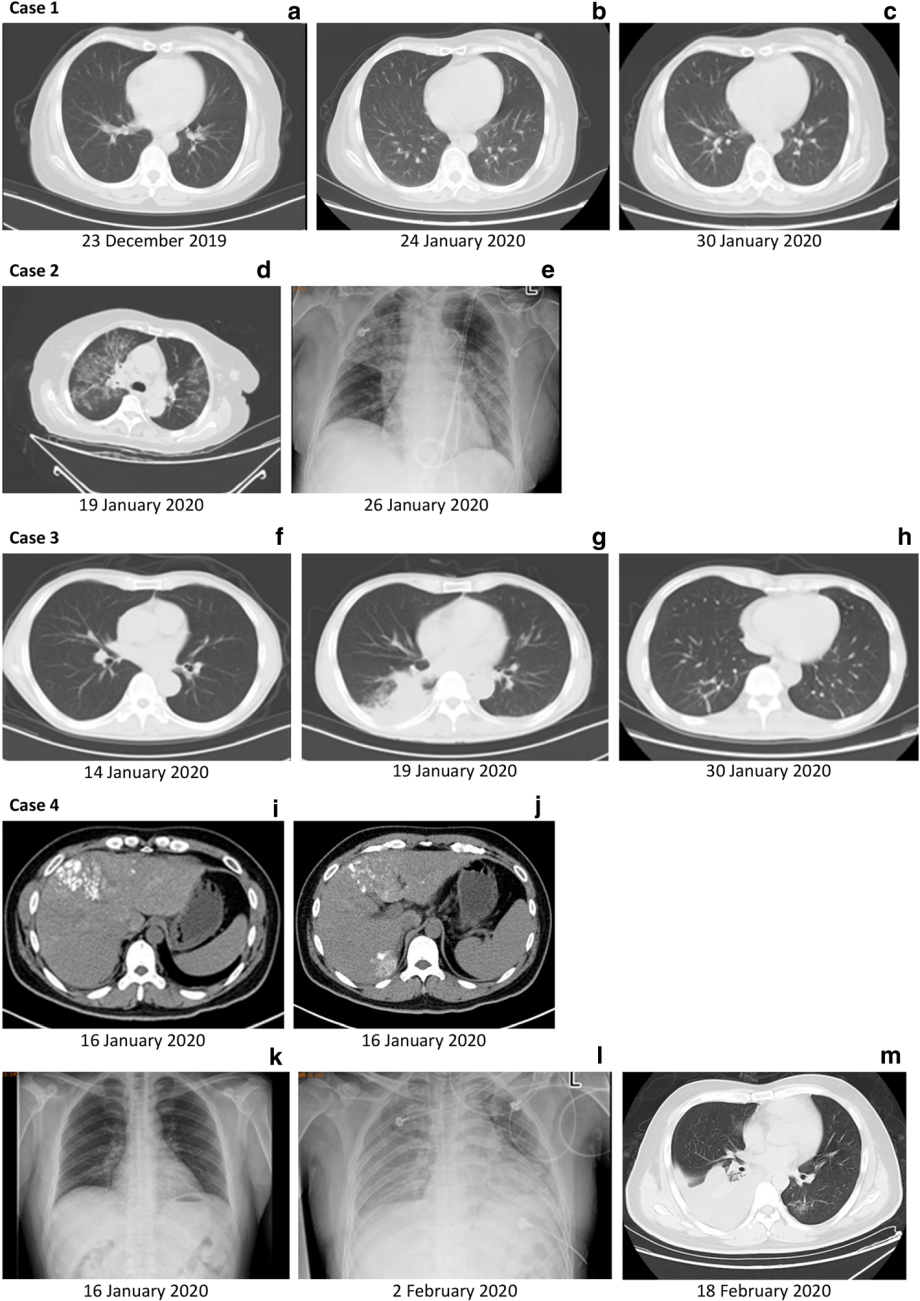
The chest X-ray/computed tomographic (CT) images of the four patients on different time points. a–c Images from Case 1. The patient had normal CT images on 23 December 2019 after admission (a). However, on 24 January 2020, after onset of fever, CT scan showed interstitial abnormalities in both lower lung, which revealed the possibility of viral pneumonia (b). And these changes were ameliorated after treatment and showed on 30 January 2020 (c). d–e Images from Case 2. The CT images showed bilateral patchy shadowing in the patient after admission (d), and the lesion progressed 1 week later (e). f–h Images from Case 3. The CT scans taken on different time points, showed local patchy shadowing in right lung 6 days after admission (g), which is the time point of fever onset in this patient. And the lesion was recovered after treatment and showed on 30 January 2020 (H). i–m Images from Case 4. i–j The CT scans showed the multiple HCC lesions in the liver after transcatheter arterial chemoembolization therapy. The X-ray examination of the patient revealed normal lung after admission (k). However, on 2 February 2020 the images from X-ray showed bilateral patchy shadowing, indicating viral pneumonia (l). The patient has been in remission of the pneumonia despite right pleural effusion was showed by CT scan on 18 February 2020 (m)
